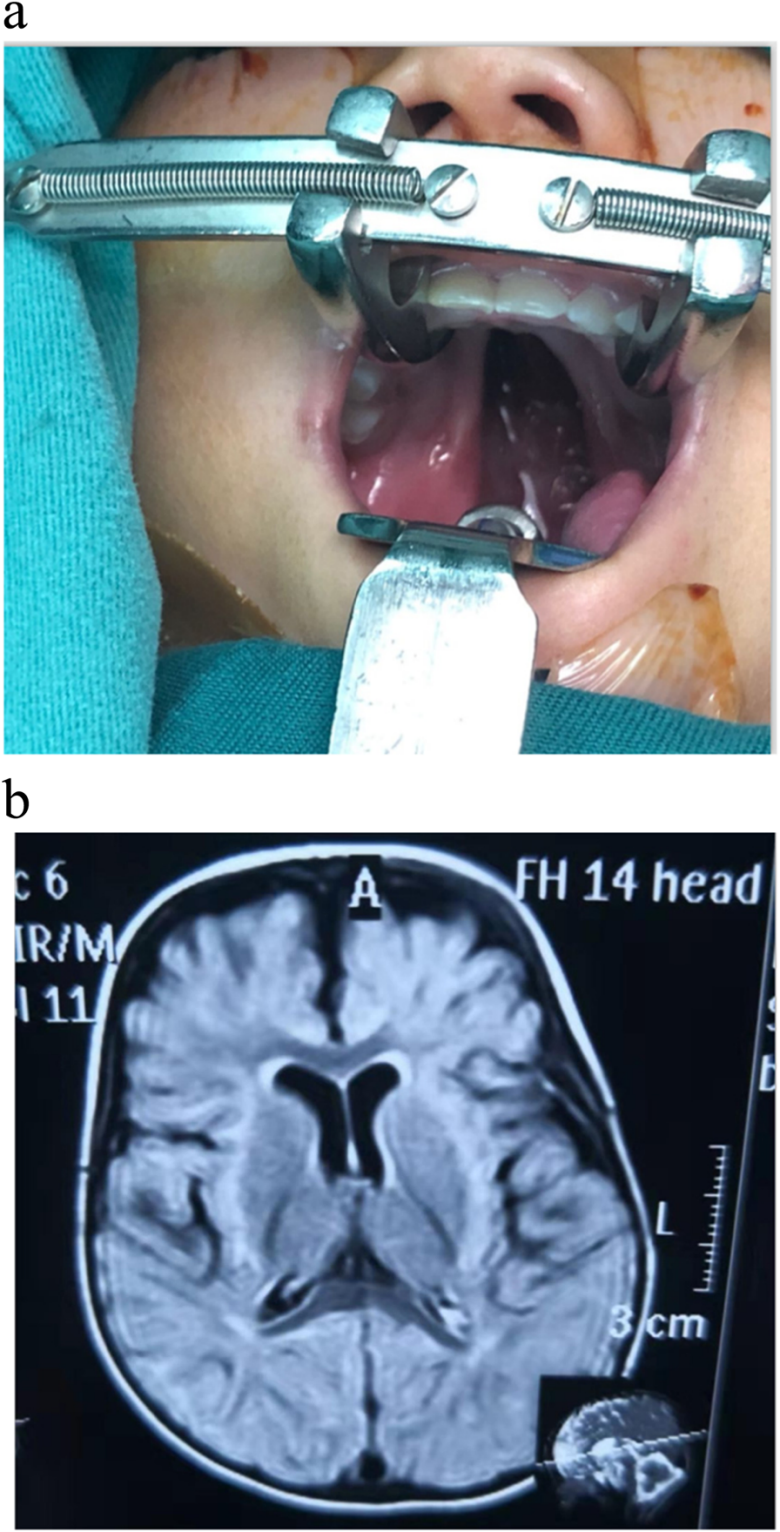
Phenotype of the patient. a The patient has a cleft palate. b MRI features of this patient
